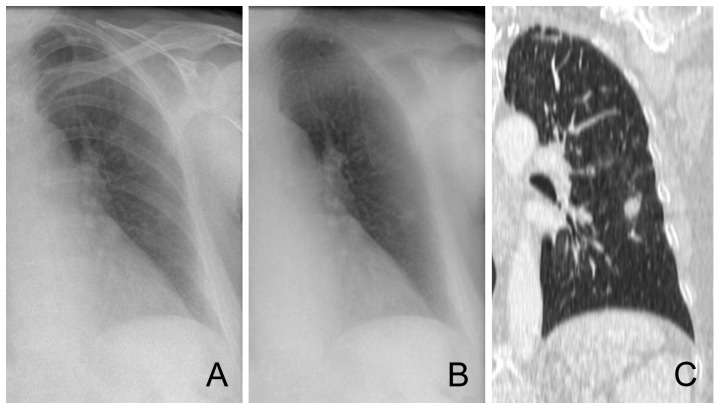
Bedside radiograph of a 58 year old female with neutropenic fever. Without BSI, 3 of the 7 observers called the radiograph suspicious, which increased to 7 observers with BSI. Original radiograph (A), bone suppressed image (B), coronal CT slice (C).
